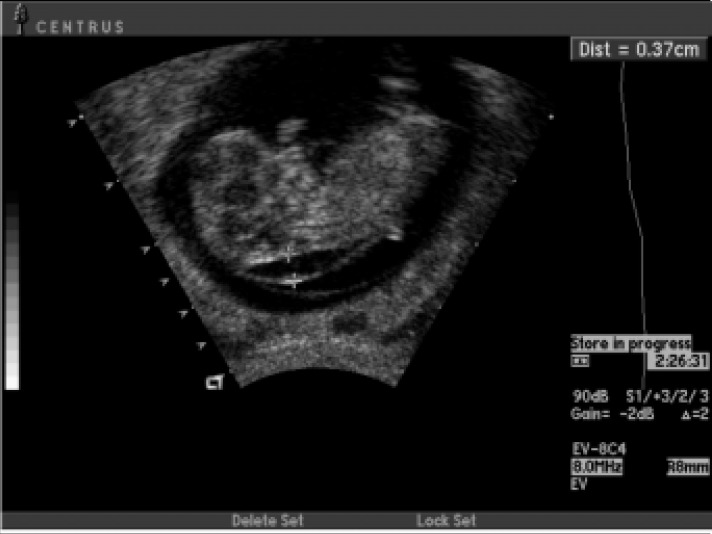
Ultrasound image of the caliper method used for measuring nuchal translucency.
